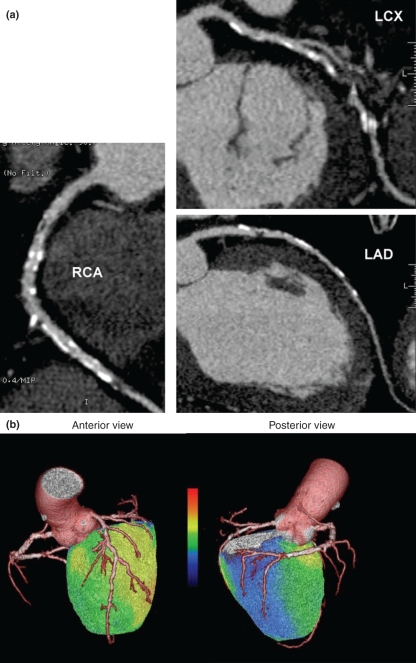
Example of hybrid PET perfusion and CT angiography. a: CT angiography (step-and-shoot mode) shows multi-vessel disease with massive coronary calcifications and severe stenoses in all major coronary arteries. b: Hybrid display during adenosine stress demonstrates quite preserved perfusion in anterolateral wall but moderate reduction in septal wall (anterior view). In RCA related region the perfusion was the most severely reduced (posterior view) indicating culprit stenosis in this vessel. Perfusion was colour scaled so that red colour denotes to 3.5 ml/min/g. Resting perfusion was normal (not shown). Total radiation dose of the hybrid study w as 11.3 mSv.
